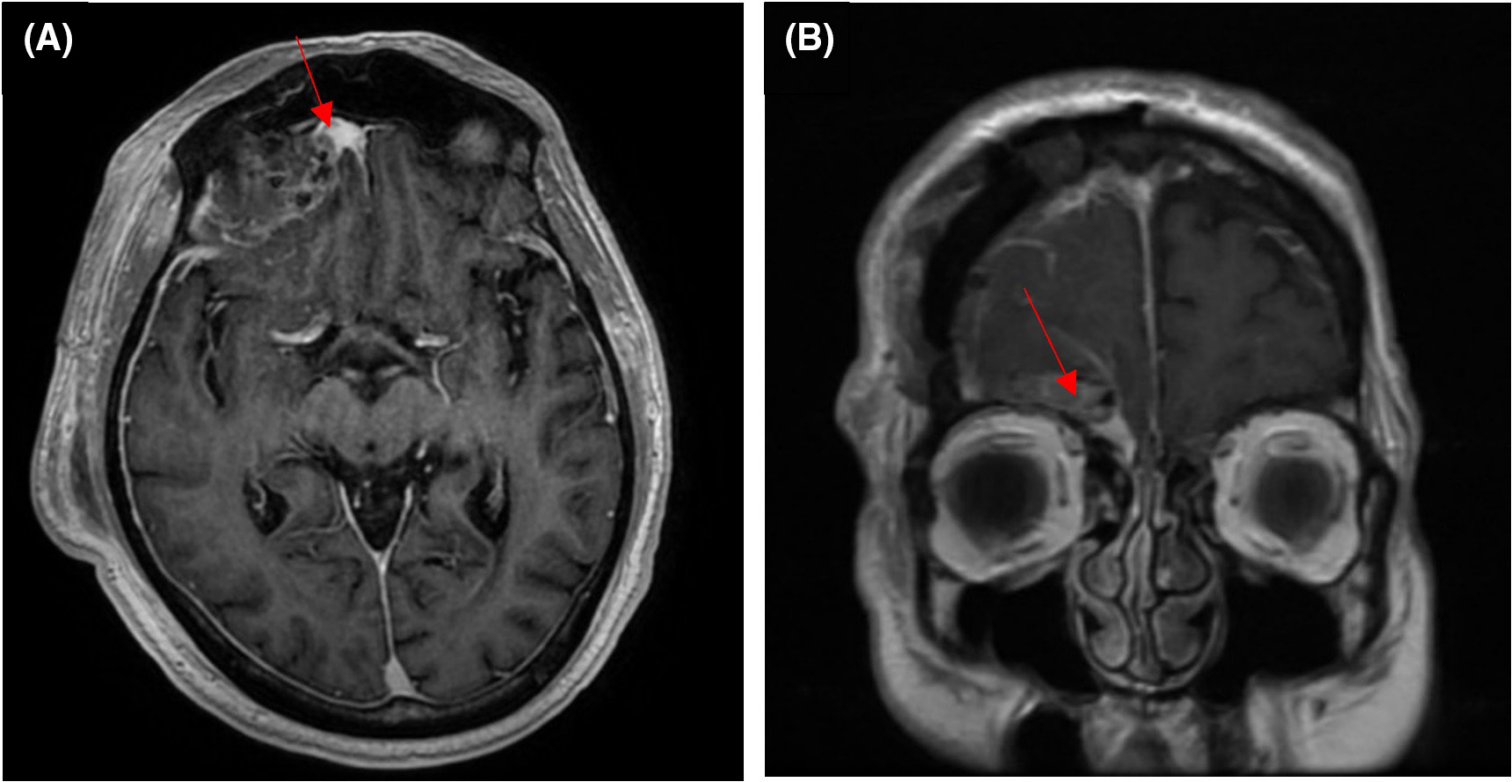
Oct 19, 2016 (Day 1 post–op). MRI head post gadolinium T2 SSFSE axial and sagittal views, showing irregular resection cavity with 2.7 × 0.9 cm band enhancing tissue along the medial supraorbital margin appears to be a residual tumor.
